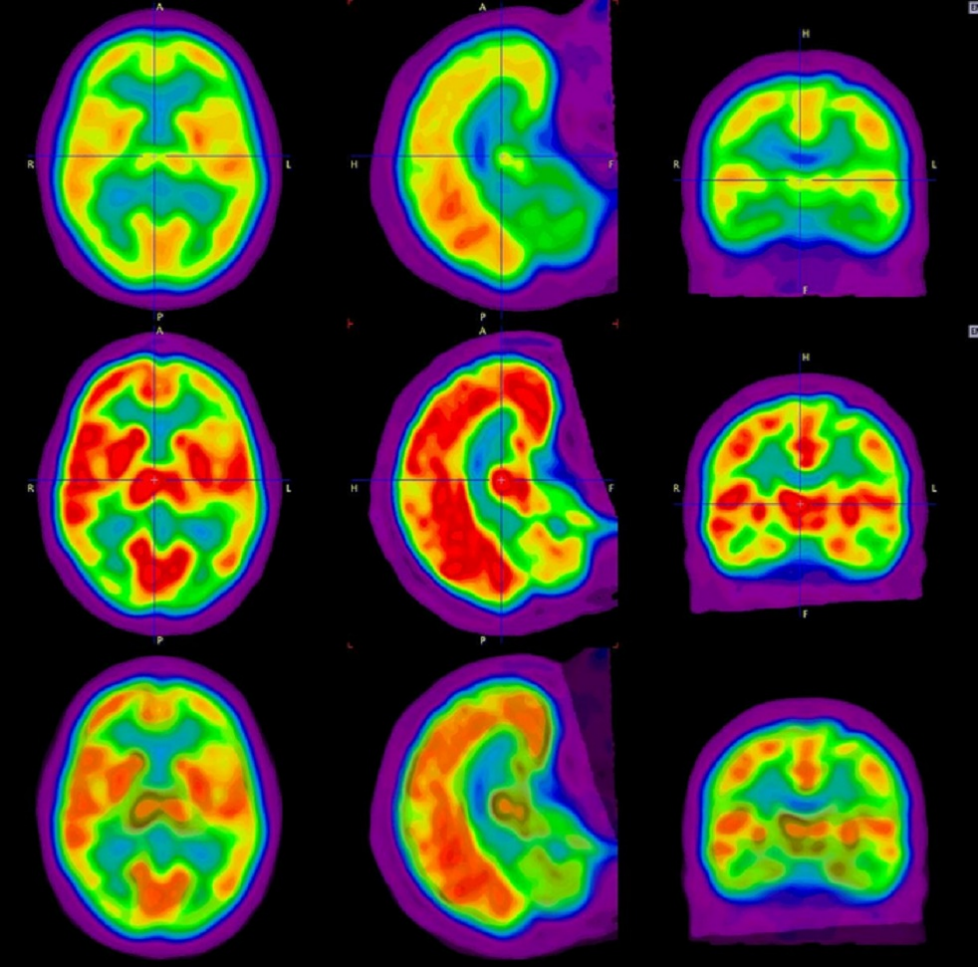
Comparison of initial and follow-up PET/CT images using PMOD/PNeuro tool The initial PET/CT demonstrates no areas of abnormal uptake to suggest brain involvement *(top row).* The subsequent PET/CT demonstrates patchy areas of intensely increased uptake *(middle row)*. Subtraction images show difference increase in uptake suggestive of lymphomatous infiltration *(third row). *The analysis performed suggested a heterogeneous pattern of uptake in the cortical gray matter and callosal white matter​, and many areas corresponded to abnormalities detected by MRI.
